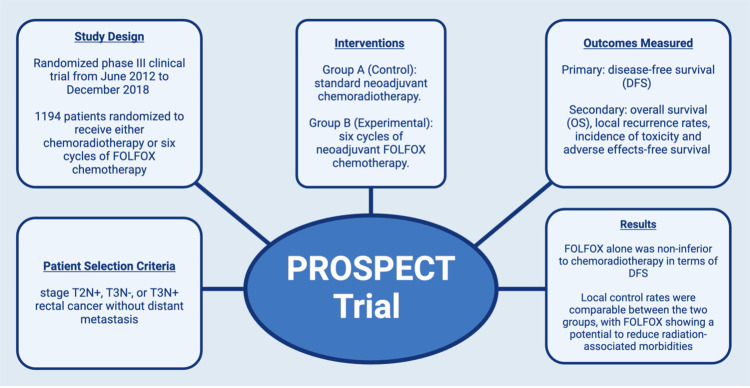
Summary of the PROSPECT trial design and outcomes demonstrating non-inferior disease-free survival with neoadjuvant FOLFOX compared to chemoradiotherapy, supporting chemotherapy-first strategies in select patients in whom radiation avoidance is clinically desirable DFS, disease-free survival; OS, overall survival This image was created using BioRender.
